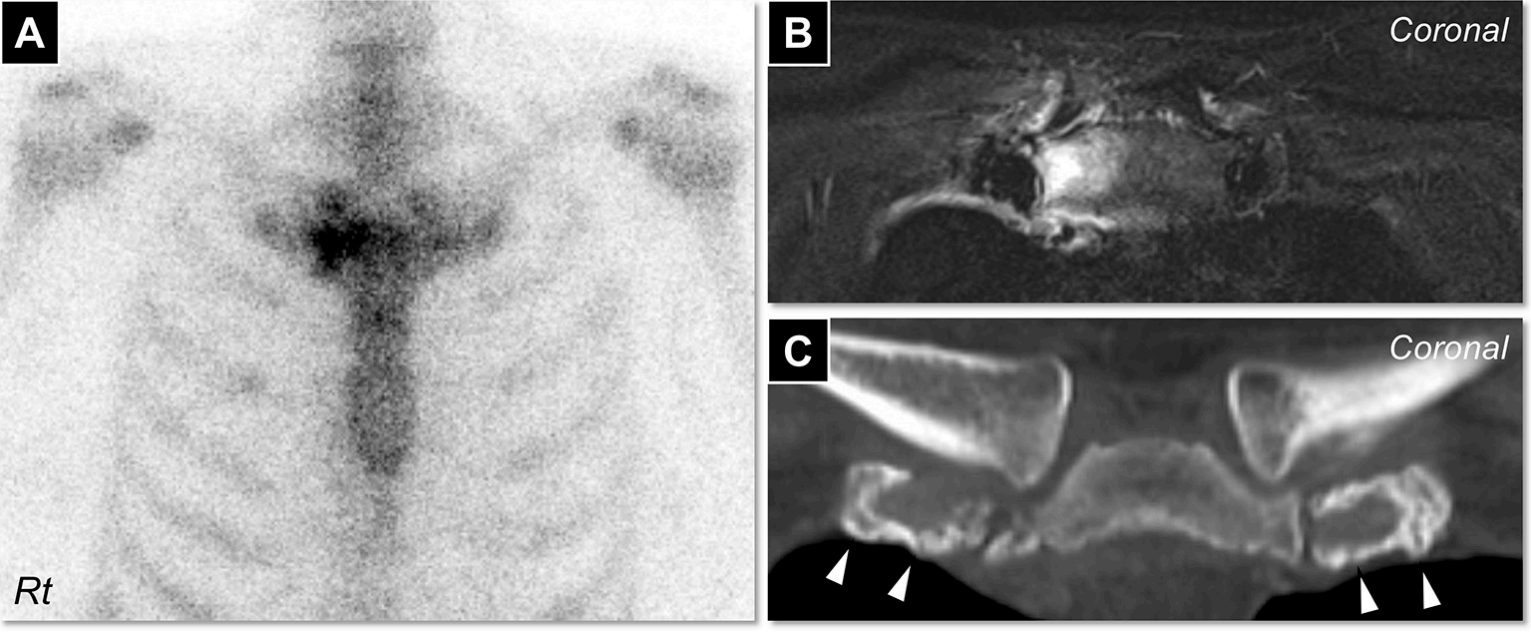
A) ^99^Tc bone scintigraphy showed “bull’s head sign.” B) Contrast-enhanced magnetic resonance imaging showed a contrast effect on the manubrium around the right sternoclavicular joint in a T2-weighted image. C) Bone computed tomography showed hyperostosis in the bilateral first sternal cartilage (arrowheads).
